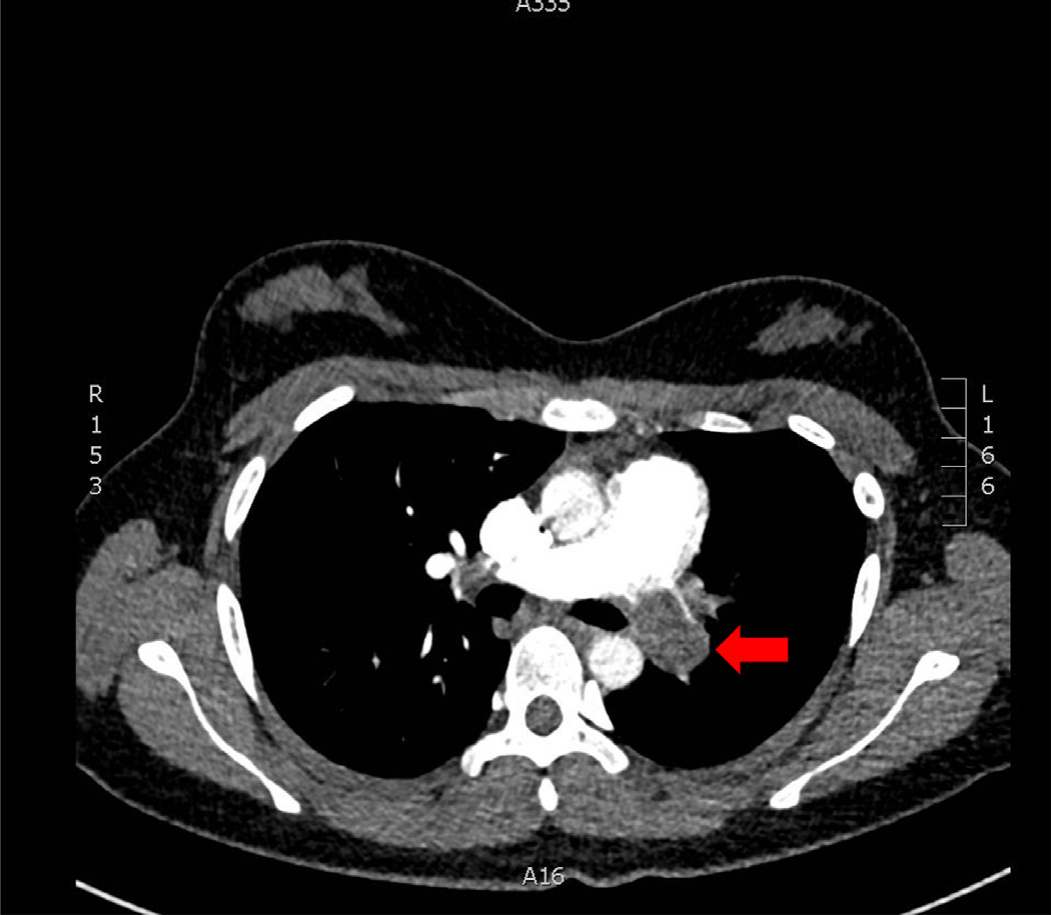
Chest computed tomography pulmonary angiogram (CTPA) showing total occlusion of the left pulmonary artery with signs of pulmonary infarction.
